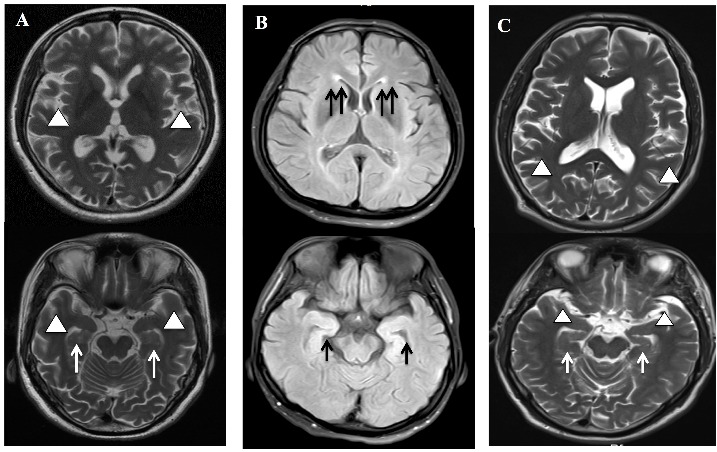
The cranial MRI of the probands in the three families A) The T2 weighed image showed atrophy of cerebral cortex (arrowhead) and hippocampus (arrow) in proband (III-1) in family 1. B) The T2 FLAIR image showed mild white matter demyelination (double arrows) in frontoparietal lobe of the proband (III-2) in family 2. C) The T2 weighed MRI image showed atrophy of cerebral cortex (arrowhead) and hippocampus (arrow) in proband (III-3) of family 3.
